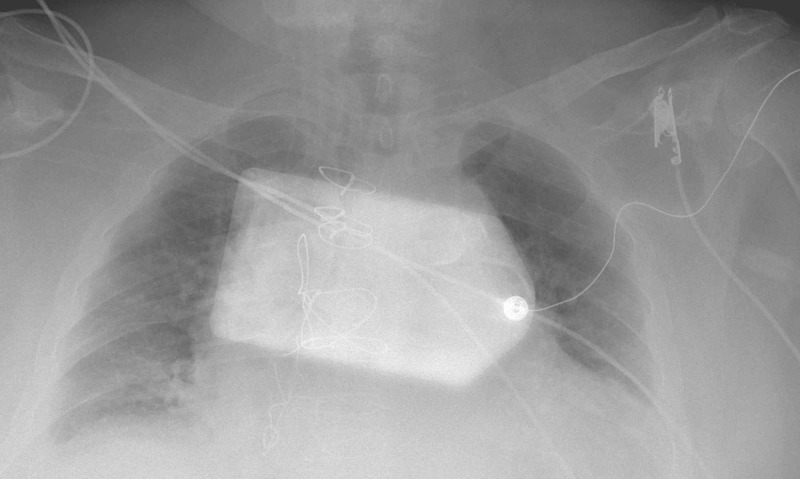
Chest X-ray single frontal view showing cardiomegaly with pulmonary venous congestion
